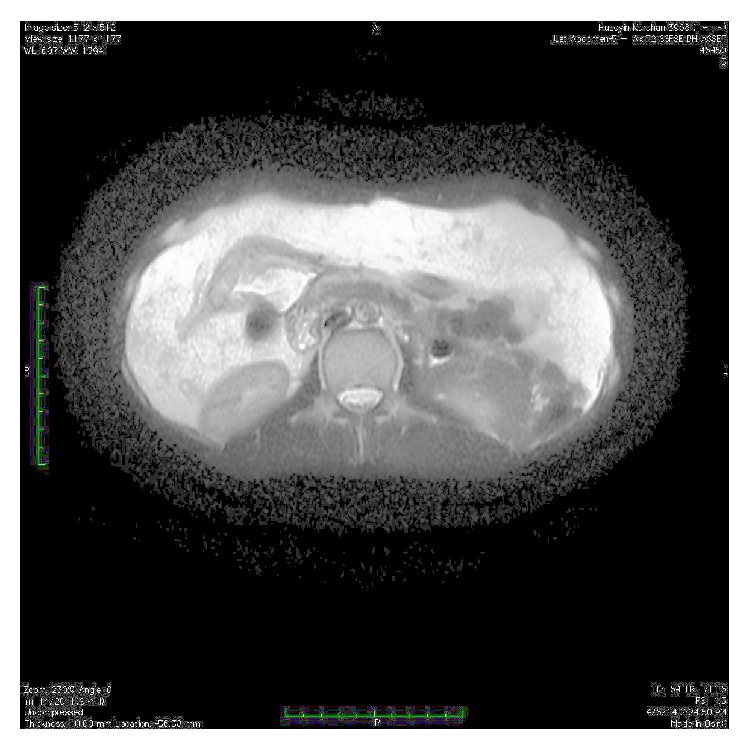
Axial T2 weighted MR imaging of abdominal cavity with diffuse abdominal lipomatosis, luminal obliteration of antrum and distal part of corpus due to lipoma and clustered intestinal loops at the right upper part of intestinal cavity.
